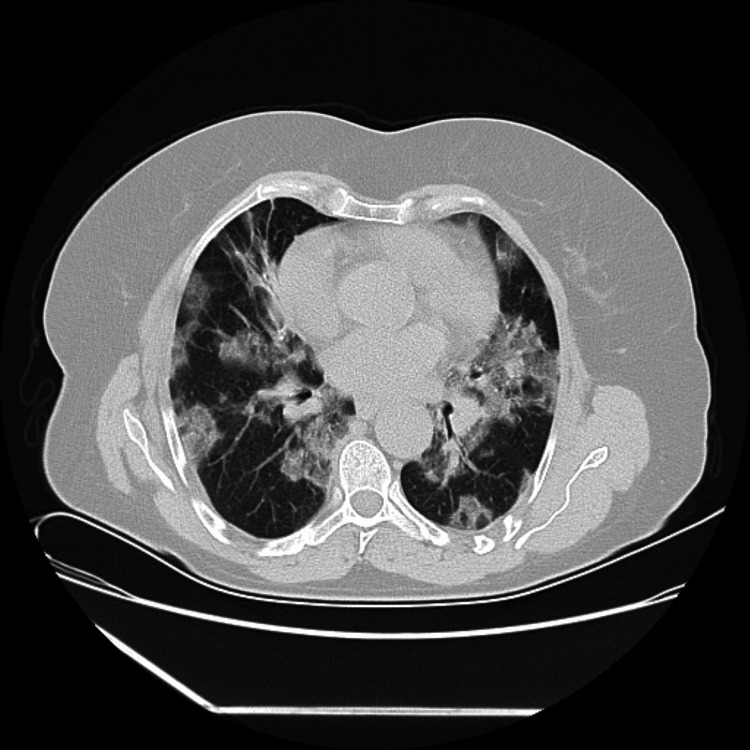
Computed tomographic scan of the thorax on the day of intensive care unit admission. Bilateral pneumonia from COVID-19, with diffuse bilateral subpleural ground glass opacities and fibrotic component.
